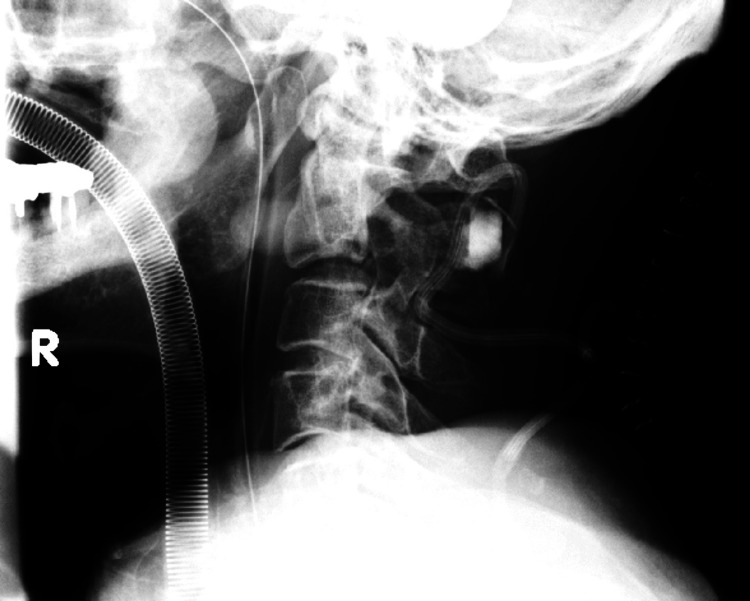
Plain lateral cervical X-ray after cervical laminoplasty showed a kyphotic angle of 10 degrees at the C2/3 level.
